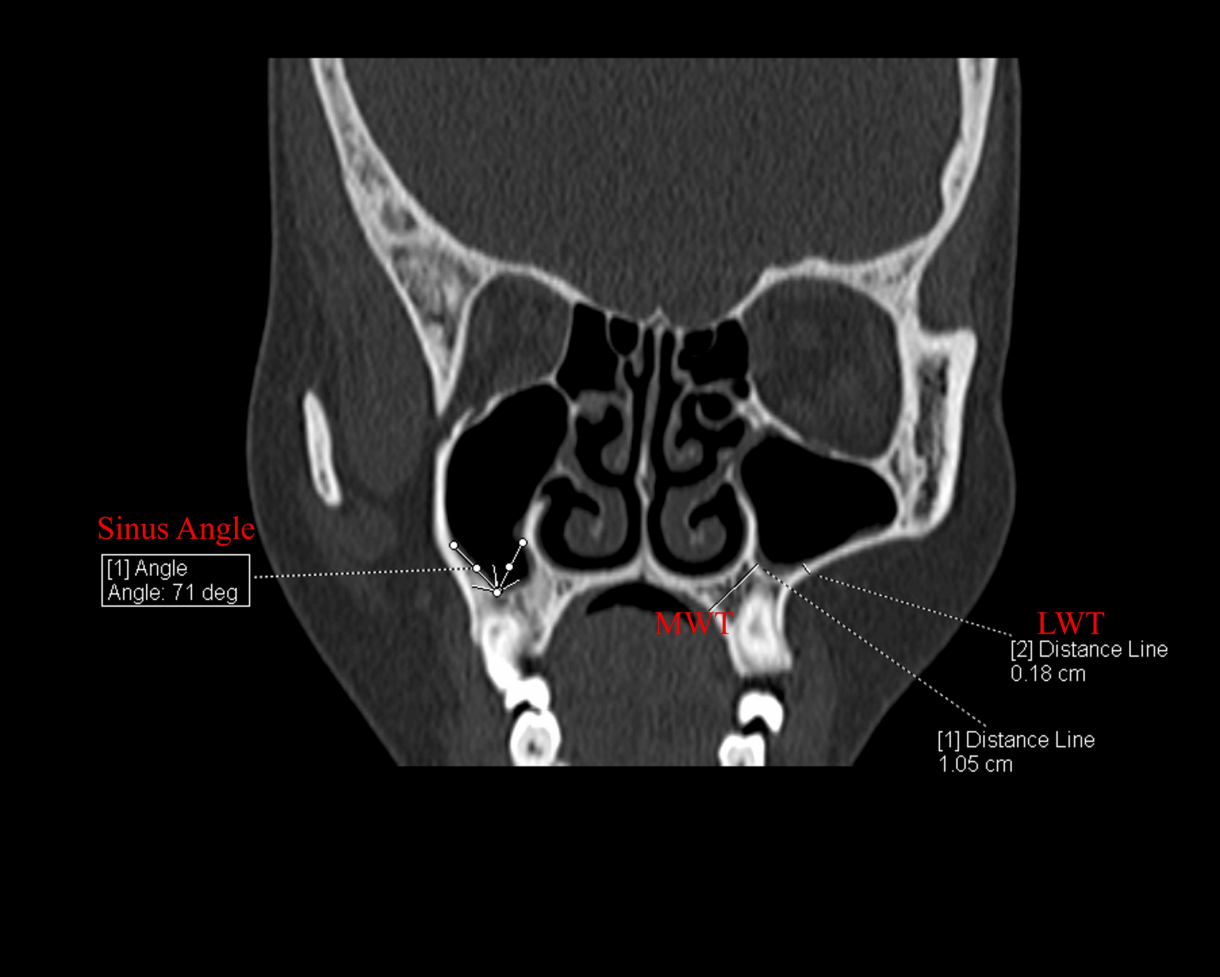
CT section showing the measurement of medial wall thickness (MWT) and lateral wall thickness (LWT) in the left maxillary sinus. Additionally, the sinus angle in the right maxillary sinus is measured in the coronal section, showing the angle formed by the intersection of the medial and lateral walls.
